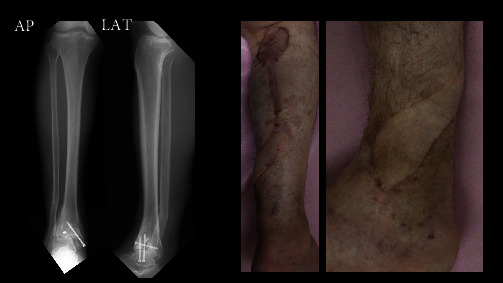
X-ray and the exterior of the RSAF one year after surgery.
